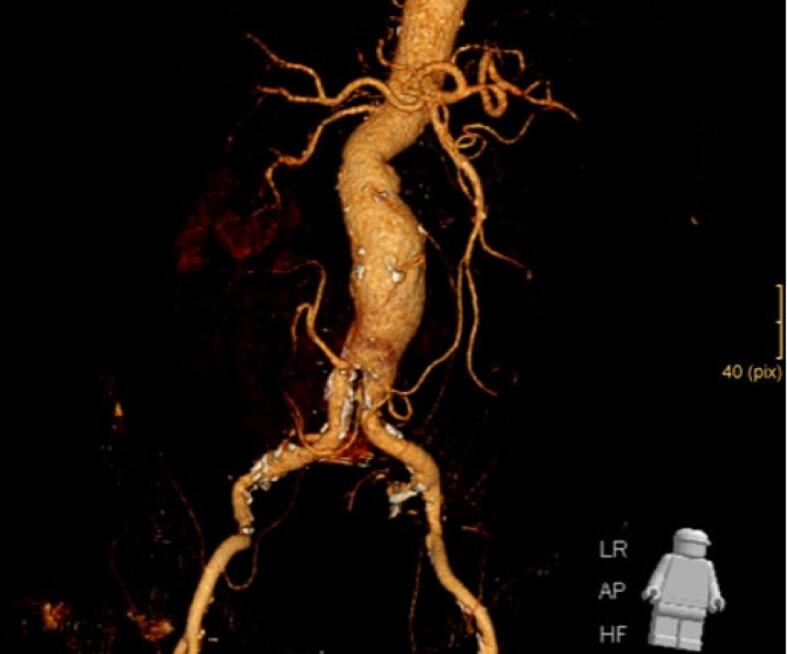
Preoperative CT angiography.
